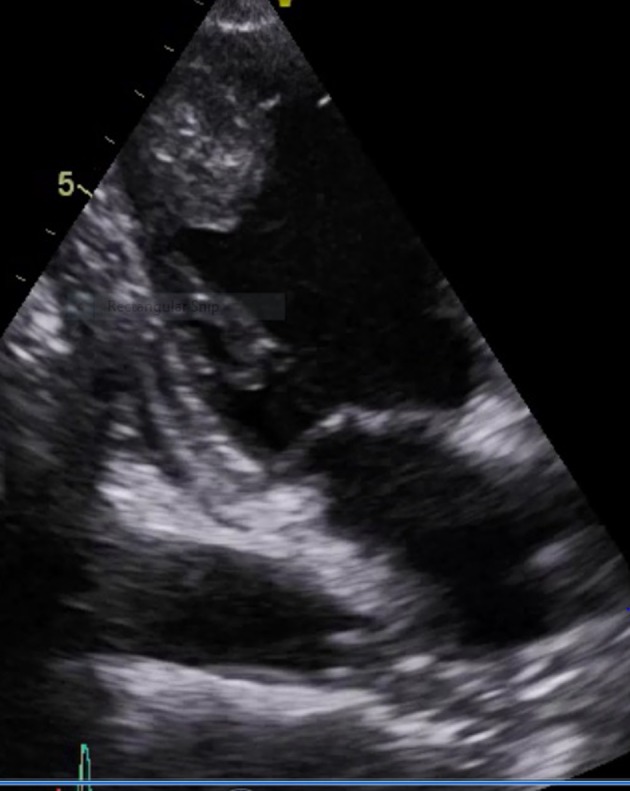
Apical four-chamber view showing heterogenous echogenic irregular vascular mass lesion in the endocardium with normal apical (left ventricular) mobility.
